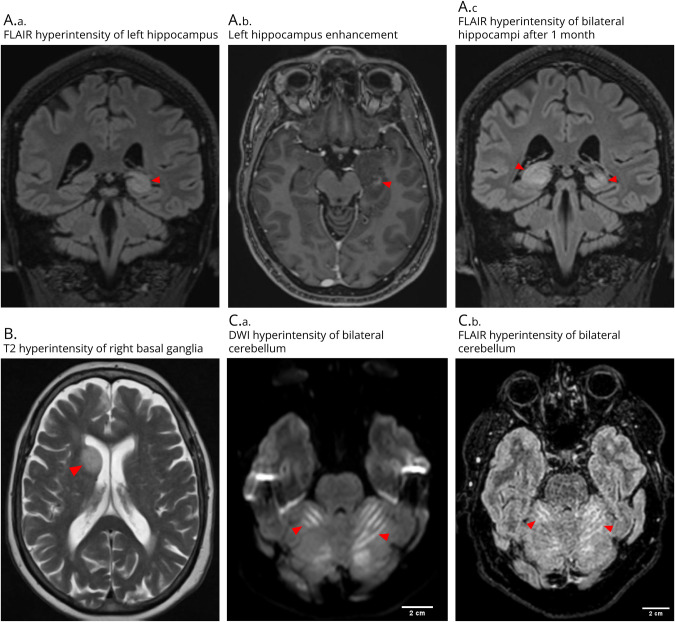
Examples of MRI Abnormalities of Anti-KLHL11 Encephalitis Patients (A.a–A.c) Patient 12: Fluid-attenuated inversion recovery (FLAIR) hyperintensity involving left hippocampus with enhancement on T1 postgadolinium, bilateral lesions were observed at follow-up MRI after 1 month. (B) Patient 14: T2 hyperintensity involving right basal ganglia. (C.a–C.b) Patient 3: Diffusion-weighted imaging (DWI) and FLAIR hyperintensity involving bilateral cerebellum. Patient numbers correspond to those in eTable 1.
